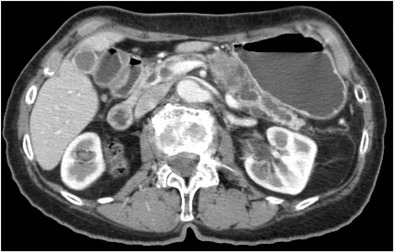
CT scan on the pancreatic lesion
